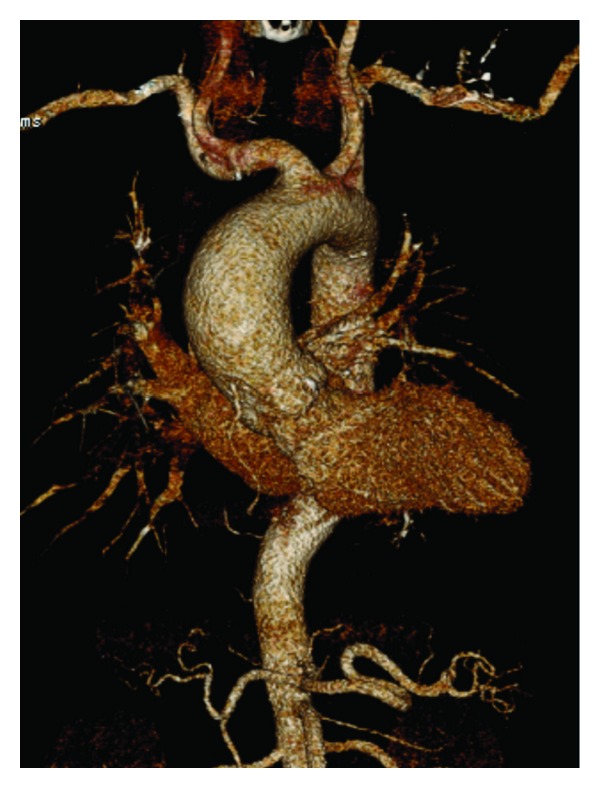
Moderately dilated ascending aorta of a young BAV patient. 3D reconstruction (CT angiography) of a typical ascending aortic aneurysm of a young BAV patient.
